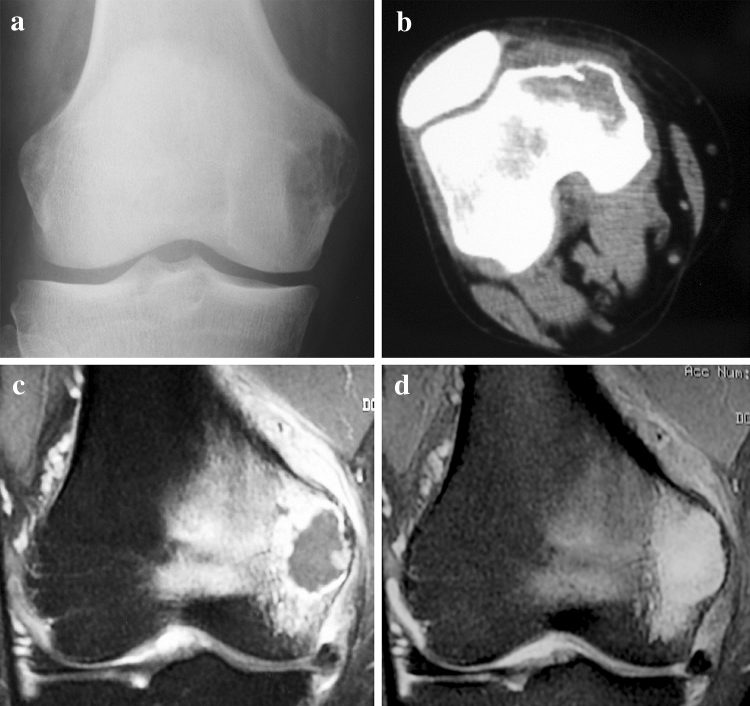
A 48-year-old man with a low-grade (grade 1) chondrosarcoma in the femur. a, b Anteroposterior radiography and computed tomography showing endosteal scalloping and the absence of calcification. c Coronal T1-weighted gadolinium-enhanced fat-suppressed magnetic resonance image showing a large area of central non-enhancement within the tumor. d Coronal T2-weighted image showing extensive bone marrow edema
